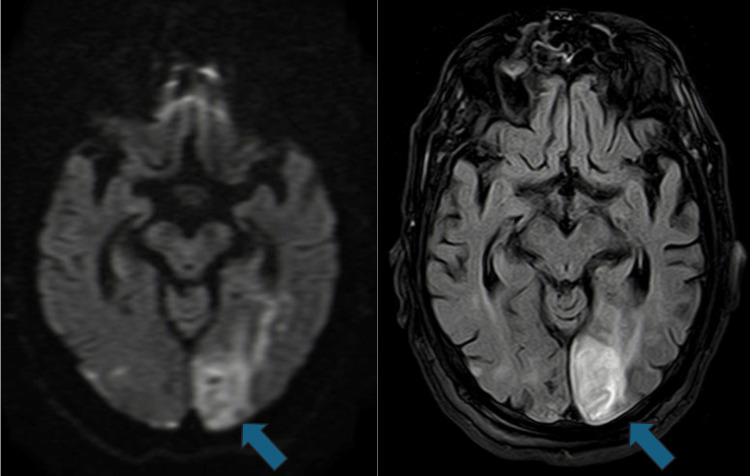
Brain MRI (DWI (left) and FLAIR (right) axial views): left occipital infarction and small contralateral occipital cortical lesions. DWI: Diffusion-Weighted Imaging, FLAIR: Fluid-Attenuated Inversion Recovery
